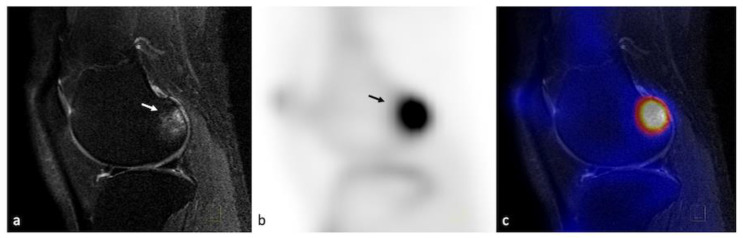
PET–MRI of the right knee of a 41-year-old female having Kellgren–Lawrence grade 2 OA, showing great signal intensity changes (arrow) in the lateral posterior femur on a T2 SPIR image (a) with a high tracer uptake on the sagittal PET image (arrow), (b) also spatially correlated on the fused PET–MRI image (c).
